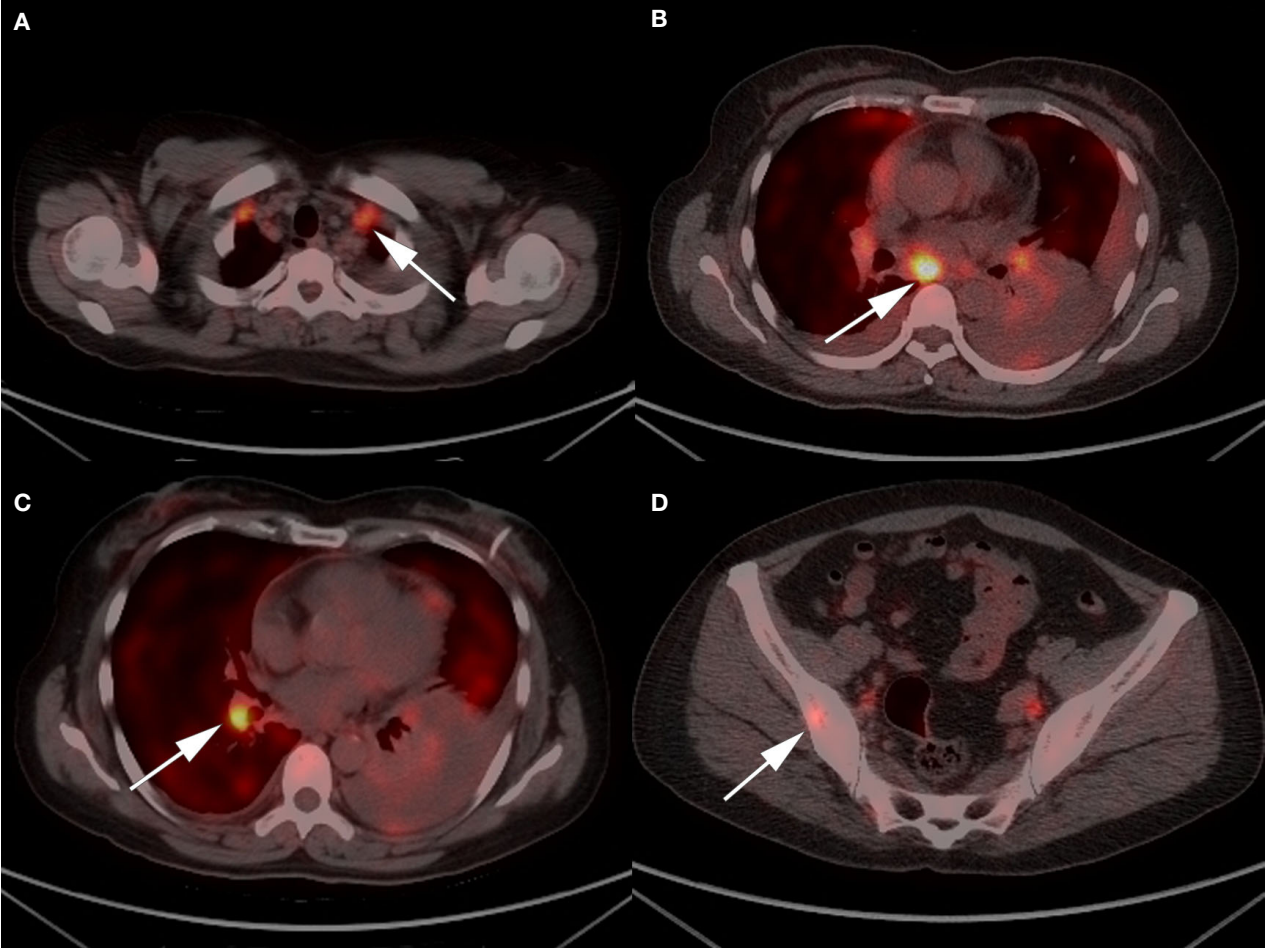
Representative ^18^F/FDG PET-CT images of the patient: (A) enlarged bilateral supraclavicular and (B) mediastinal lymph nodes; (C) a 3.4*2.4 cm soft tissue density mass in the right lower lobe; and (D) increased metabolism in right ilium.
